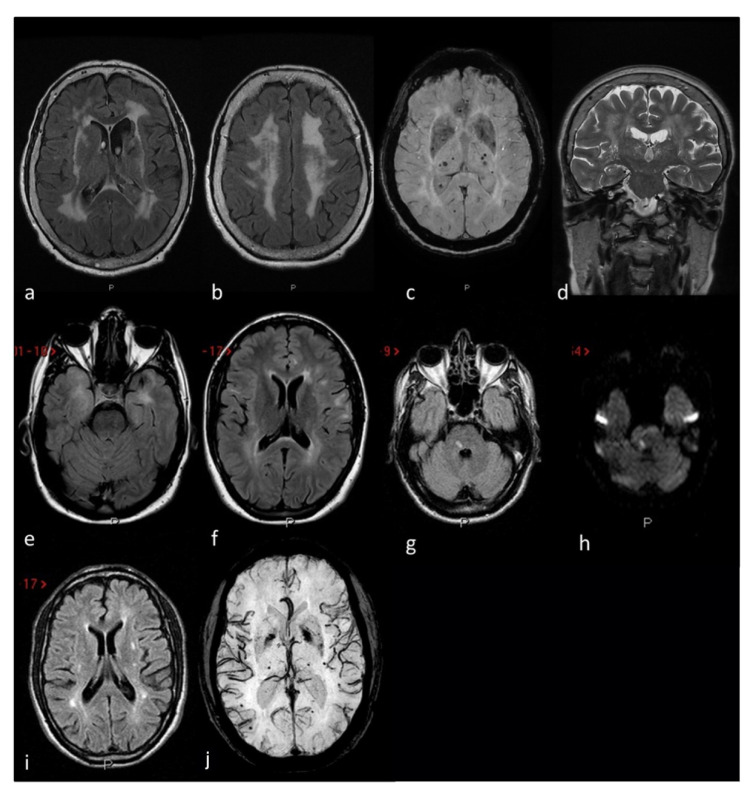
MRI markers of small vessel disease in three CADASIL patients: Patient 1 (a–d) severe leukoaraiosis involving subcortical white matter, external capsule and periventricular regions in a symmetrical pattern on axial FLAIR (a,b) MRI, associated with deel and lobar supratentorial microbleeds on SWI (axial MiP sequences) MRI (c) and enlarged perivascular spaces in the basal ganglia on coronal T2W (d) MRI. Patient 2 (e,f) anterior temporal lobe involvement (e) and periventricular and iuxtacortical white matter hyperintensities (f) on axial FLAIR MRI. Patient 3 (g–j) recent subcortical ischemic stroke on the right portion of the pons on FLAIR (g) and DWI (h) MRI sequences, supratentorial white matter hyperintensities with a dotted distribution involving the external capsule and the periventricular regions on axial FLAIR (i) MRI and multiple supratentorial deep and lobar microbleeds on SWI (MiP sequences) MRI (j).
